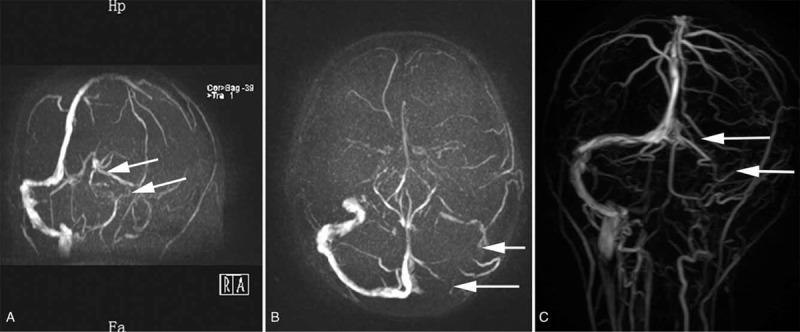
T2-weighted MRI at the 20-day follow-up show residual cerebellar infarctions in the left frontal (A) and temporal (B) lobes (arrows). MRI = magnetic resonance imaging.
